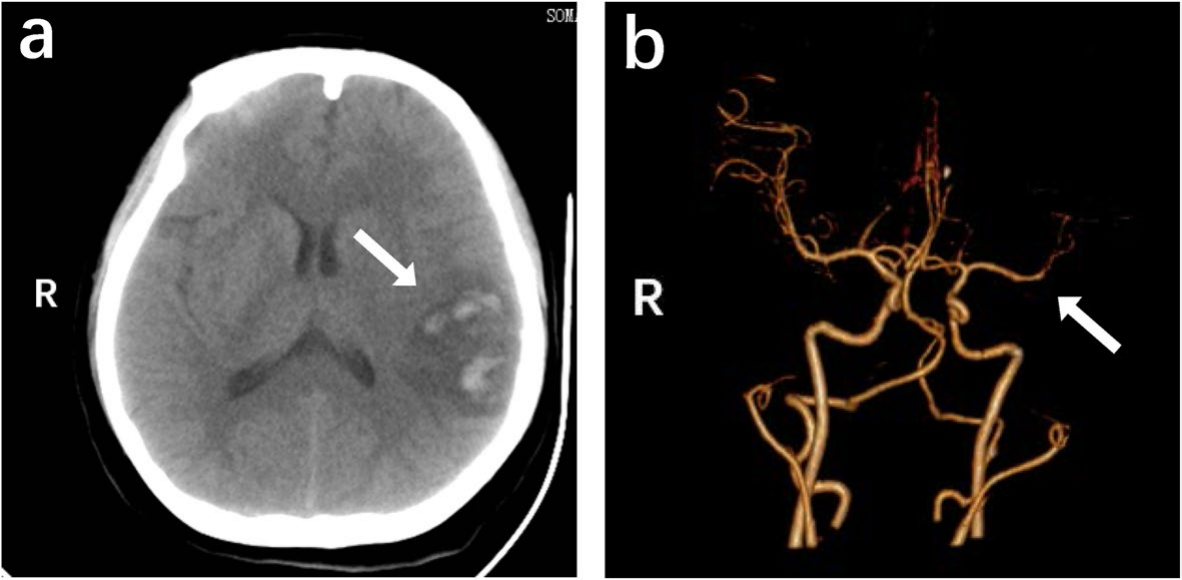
CT/CTA images on admission. a Typical haemorrhagic infarction findings detected on CT. b The distal left middle cerebral artery appeared vague on CT angiography
